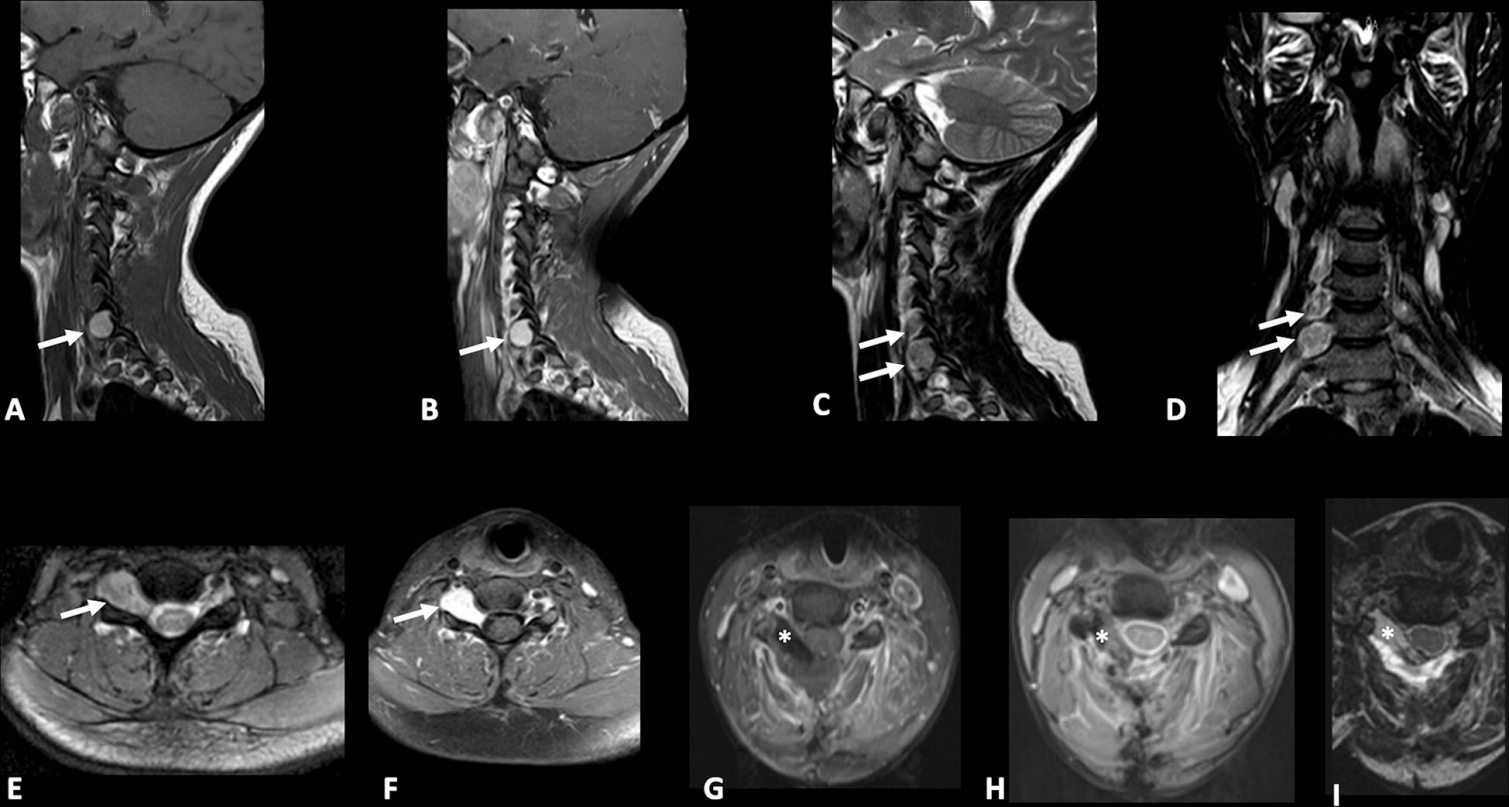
Pre-operative sagittal T1-weighted (A), sagittal (B) and axial (F) post-gadolinium T1-weighted, sagittal (C), coronal (D), axial (E) T2-weighted Nuclear Magnetic Resonance (MRI) images demonstrating the presence of two right sided extradural spinal lesions (arrows) at C5-C7 levels one of which is larger with intraforaminal and paravertebral extension and “dumbbell” shape. Postoperative axial post-gadolinium T1-weighted and fat suppression (G), Gradient echo (GRE) (H), and T2-weighted (I) MRI images demonstrating gross total resection (GTR) of lesions and placement of fat tissue (asterisk) to reinforce dural closure.
